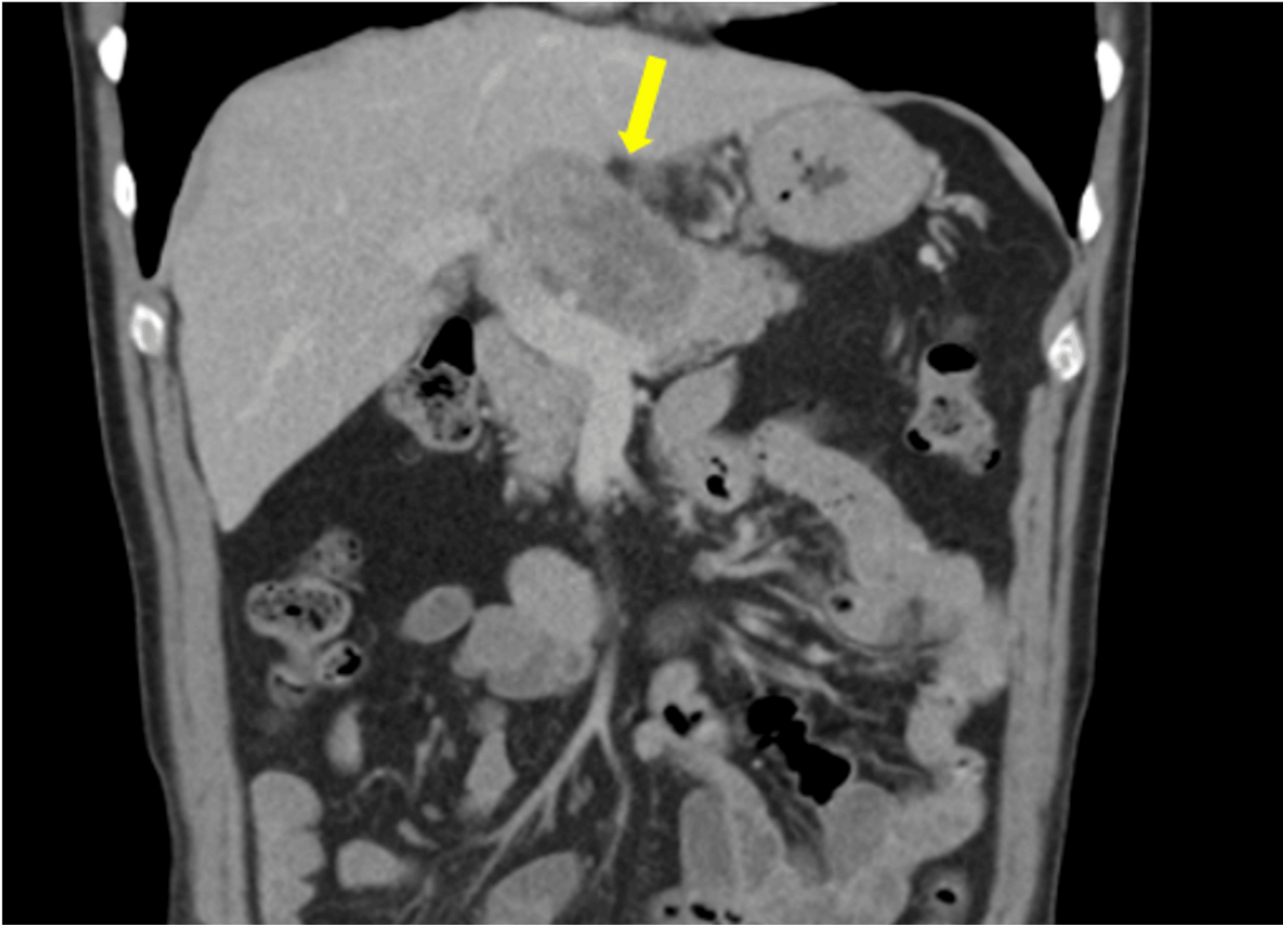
Coronal CT image at presentation demonstrating the primary tumour in the pancreatic neck (yellow arrow) encasing the hepatic artery, consistent with locally advanced disease.
